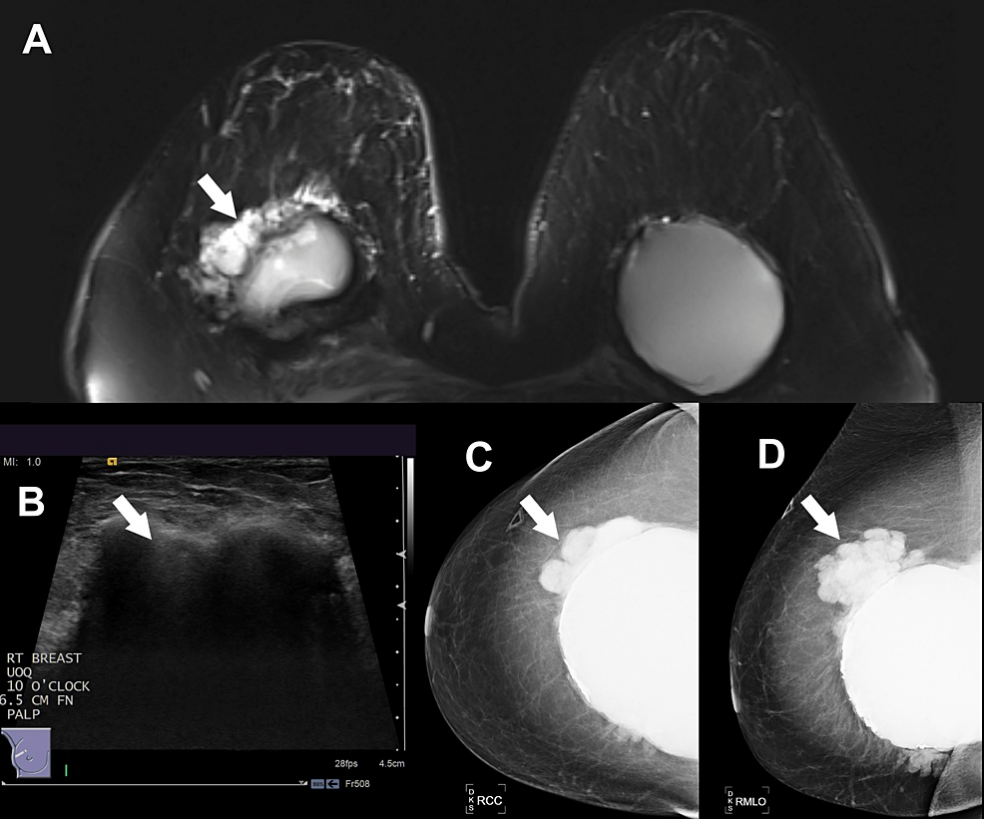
(A) Magnetic resonance imaging showing heterogeneous, lobular pericapsular enhancement at the 10 o’clock position 6.5 cm from the nipple, measuring 45 × 70 × 50 mm. (B) Ultrasound demonstrating a snowstorm appearance in the same location. (C) Craniocaudal and (D) mediolateral oblique mammography views showing extracapsular silicone rupture and medial herniation.
